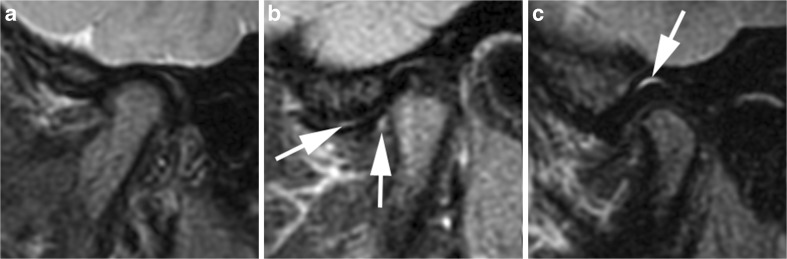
No joint effusion (grade 0). a-c Sagittal oblique fat-saturated T2-weighted fast spin echo images (TR/TE 5,400/77 ms). There is no high signal intensity fluid within the joint compartments in a 3-year-old boy (a). There is a normal amount of fluid in the anterior recesses (arrows in b) in a 14-year-old boy and in the superior joint compartment (arrow in c) in a 14-year-old girl
